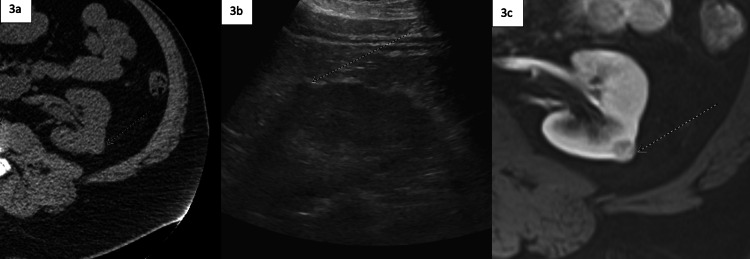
Imaging studies showing workup of indeterminate renal lesion 3a: Unenhanced CT demonstrating an indeterminate density left renal lesions. Note the small size of the lesion and the significant intra-abdominal and subcutaneous adipose tissue. The reading radiologist recommended an ultrasound for characterization. 3b: Renal ultrasound in a poor candidate, upon which the lesion was not able to be definitively characterized as cystic or solid, necessitating the ultimate recommendation of an abdominal MRI. 3c: Contrast-enhanced MRI characterizing the lesion as a small, enhancing malignancy.
